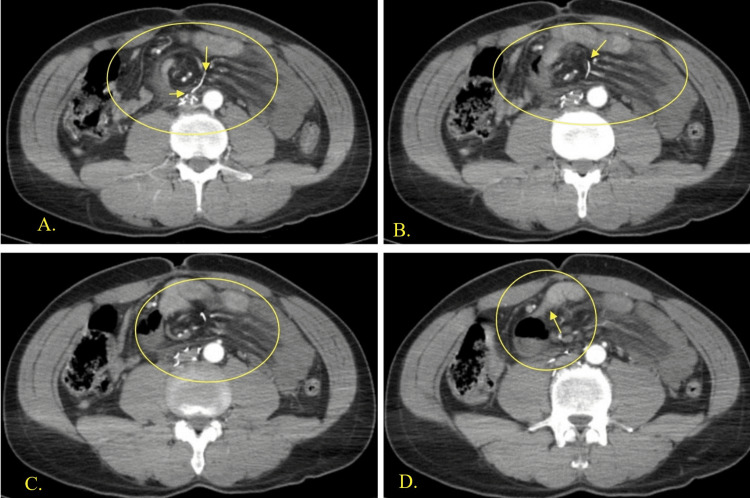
Axial views of CT images of the abdomen with IV contrast Images 3A-3D show an IVC filter wire strut measuring 25 mm penetrating the inferior vena cava into the peritoneal cavity, with progression of mesenteric swirl. Image 3A shows the beginning of wire strut penetration through inferior vena cava. Image 3B shows a piece of IVC filter wire broken off and in the mesentery of the small bowel, creating a mesentery swirl with tunneling. Image 3C shows progression the swirl with bowel wall changes. Image 3D shows the transition point in the obstruction CT, computed tomography; IV, intravenous; IVC, inferior vena cava
